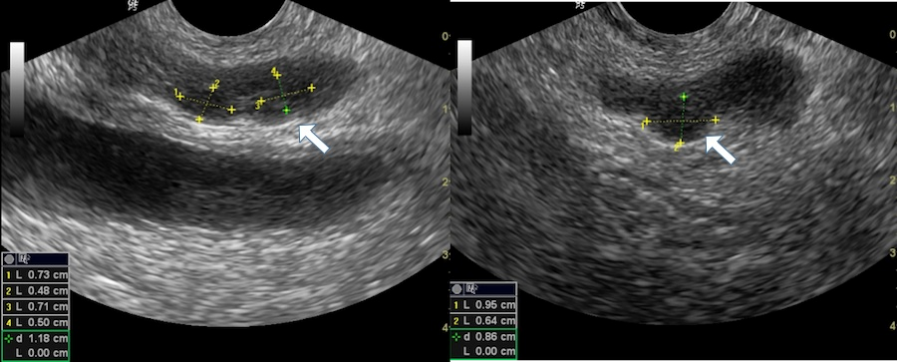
Transrectal ultrasonogram axial images The right and left images demonstrate multiple well-defined rounded hypoechoic lesions with echogenic debri within suggestive of left-sided seminal vesicle cysts with debris; the right-sided seminal vesicle is not visualised and is likely hypoplastic.
